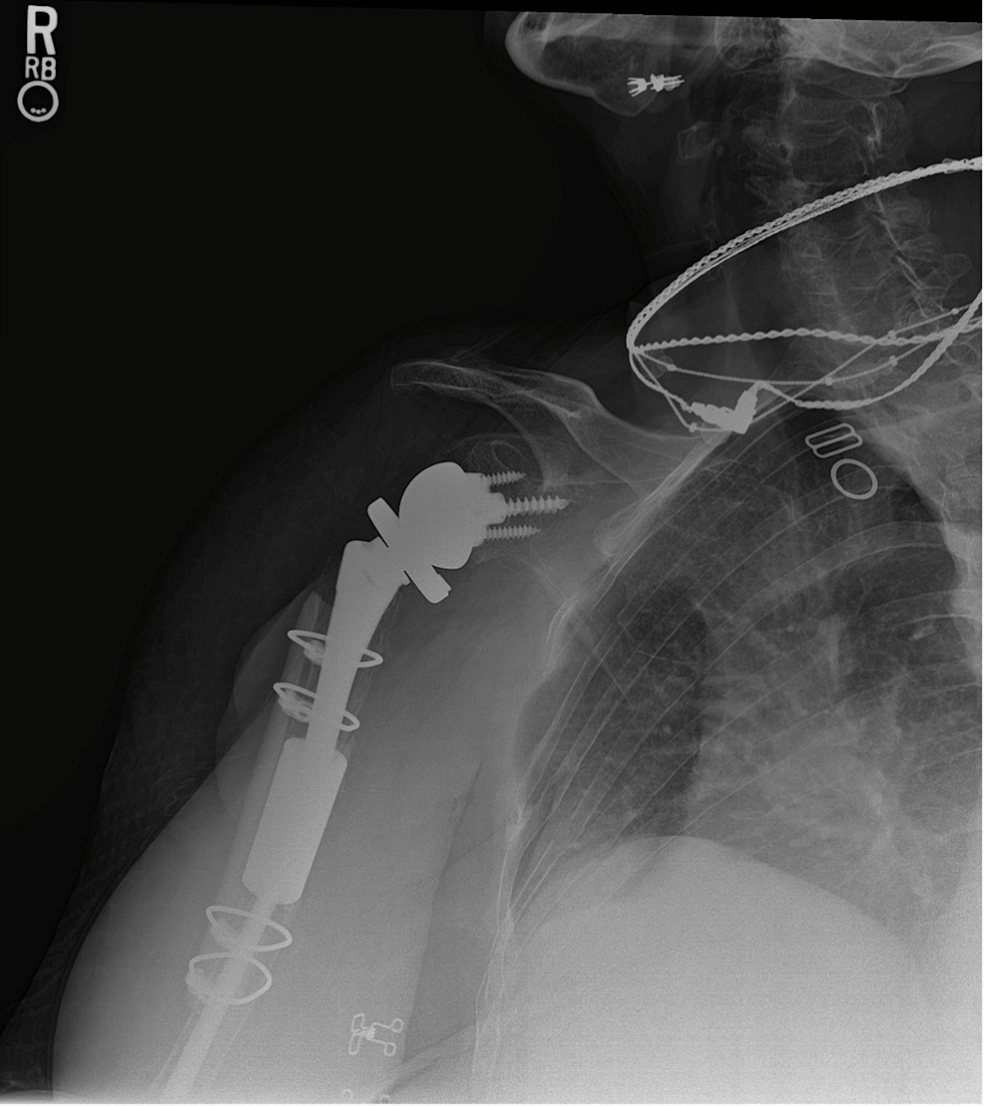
AP radiograph of the right shoulder at six-month follow-up. AP: anteroposterior
